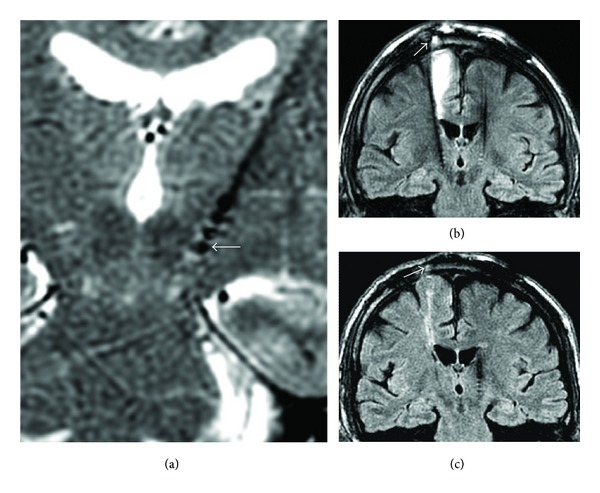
(a) Coronal 3D long echo train T2 image showing 2.2 mm tip diameter (ETL 80, TE 85 ms) with visualization of white matter tissue adequately; (b) and (c) 3D long echo train FLAIR MR signal for an infected DBS lead (arrow showing infection prior to removal (b) and after removal and treatment (c)). Note the artifactual bright dots in T2 images are not prominently present on FLAIR while the size of the implant lead is similar to those in T2 images (Figure 1).
